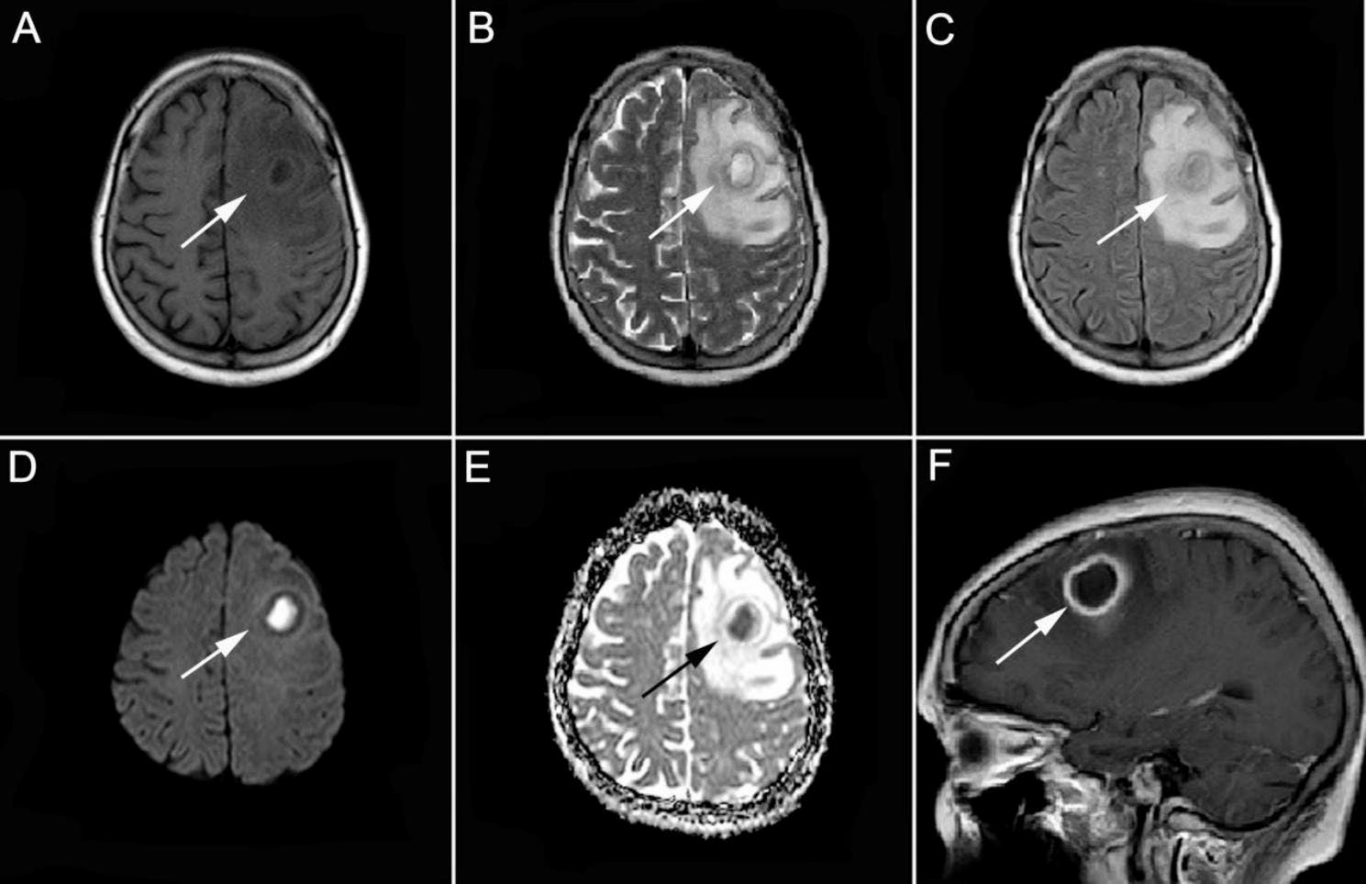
1.5-T MRI of brain abscesses before surgery. Typical imaging manifestations of brain abscesses were found in the left frontal and parietal region on coronal MRI slices with hypointensity on T1-weighted imaging (A) showing encephaledema. Hyperintensity on T2-weighted imaging (B), fluid-attenuation inversion recovery (FLAIR) imaging (C), and diffusion-weighted imaging (DWI) (D), as well as hypointensity on apparent diffusion coefficient (ADC) (E) imaging with encephaledema were also observed in brain abscesses—frontal and parietal lesions. Brain abscesses showed thin-rim enhancement with encephaledema on sagittal slices of MRI (F). White and black arrows indicate lesions
